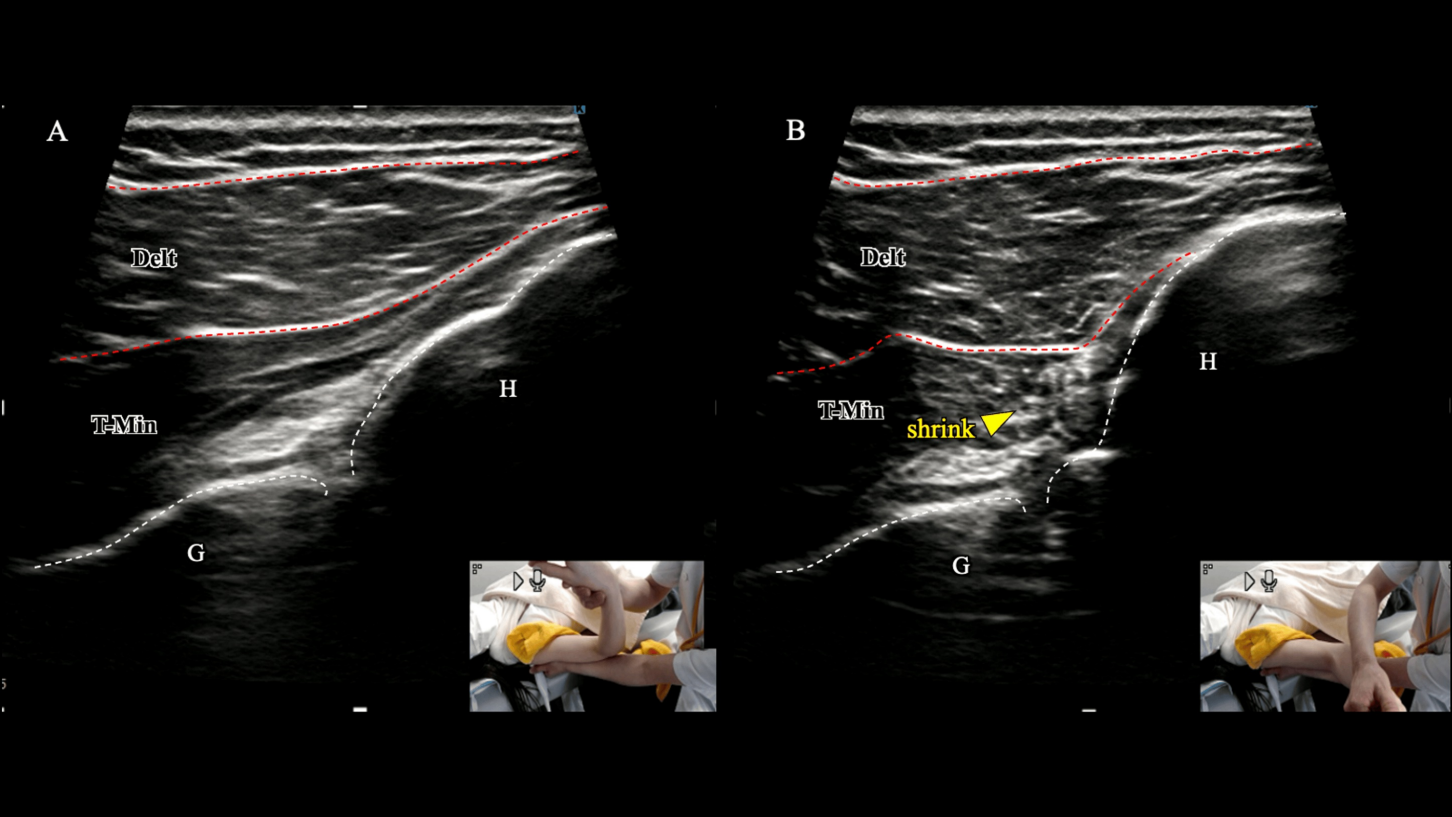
Ultrasonographic assessment of teres minor dynamics during external rotation (ER). During active-assisted ER from the start (A) to end range (B), the teres minor (T-Min) shows localized tissue folding/buckling with reduced excursion along the posterior glenohumeral joint, coinciding with the patient’s stiffness and perceived weakness. T-Min, teres minor; Delt, deltoid; G, glenoid; H, humeral head
